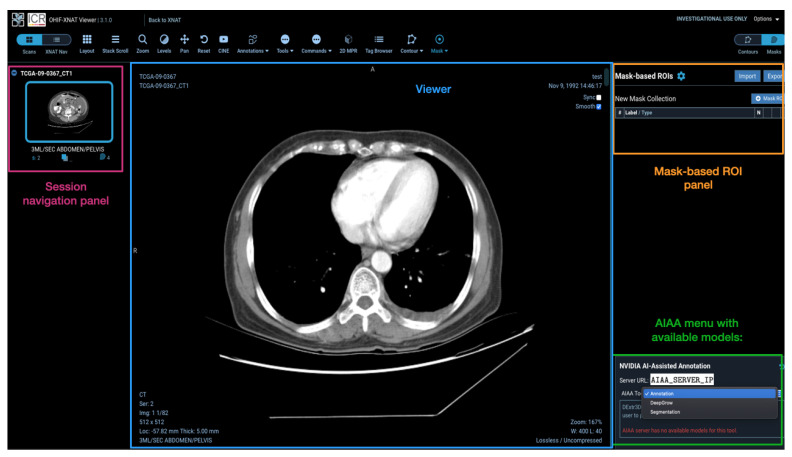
View of a CT imaging session in the OHIF viewer plugin to XNAT with the mask menu on the right-hand side presenting the connection to the AIAA server established and the drop-down menu with the categories of available AI models.
